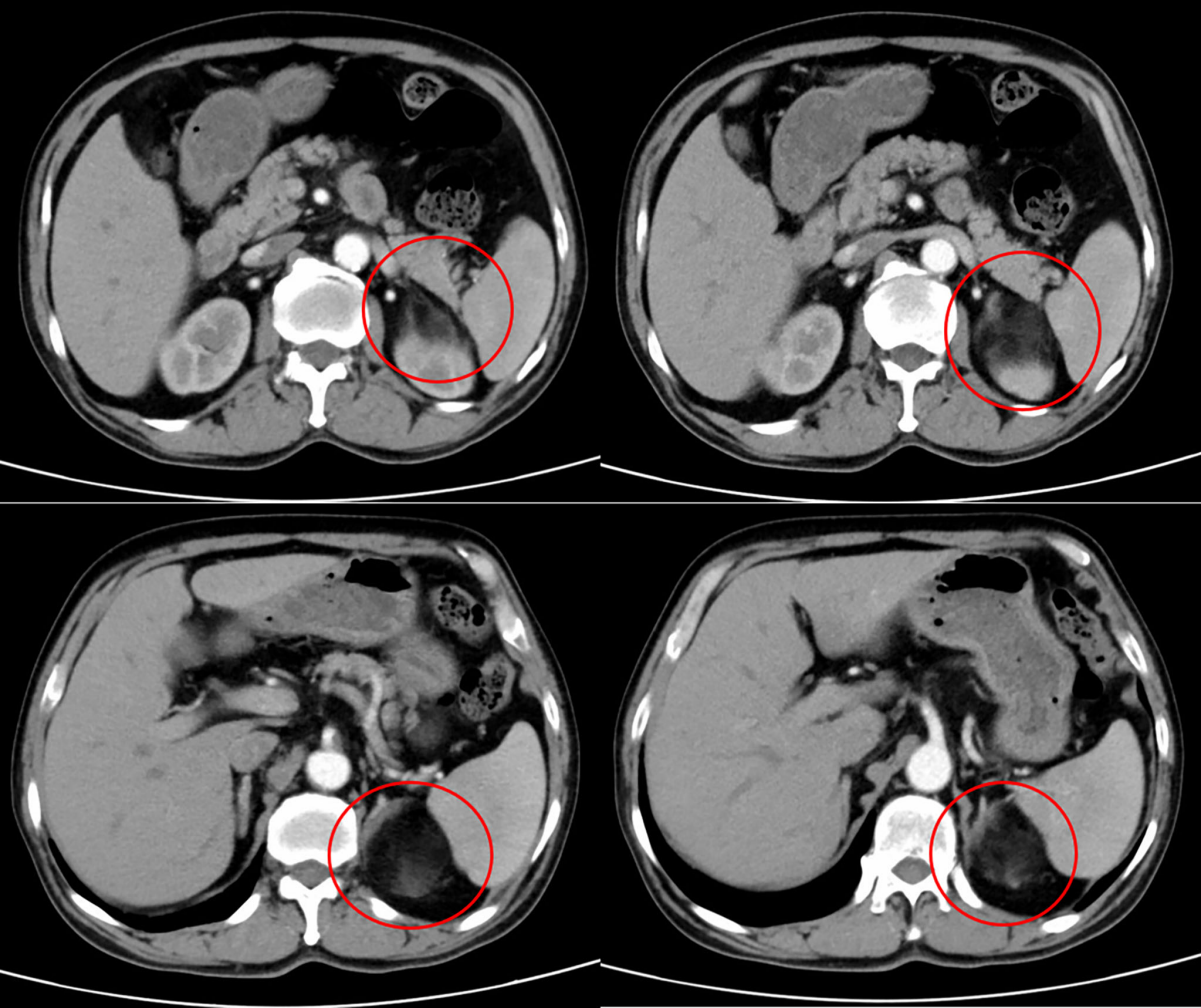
Abdominal computed tomography imaging, representing a left adrenal mass of 5.1×5.1cm.
